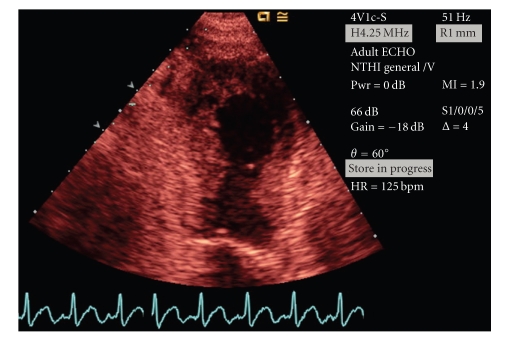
Left ventricular apical akinesia and ballooning visualized during systole on echocardiography.
